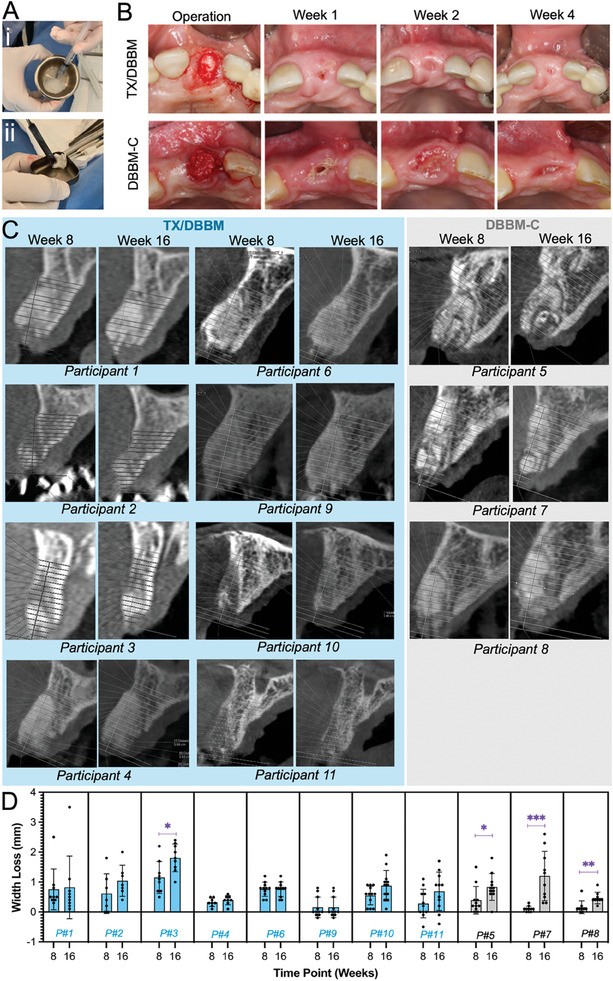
Clinical study of TX/DBBM in ARP post tooth extraction. A) TX/DBBM sample preparation before the operation: i) mixing TX hydrogel with DBBM, and ii) forming a moldable and cohesive putty of TX/DBBM. B) Treatment site at operation (Day 0), and Week 1, 2, and 4 post‐operations of participants with TX/DBBM (TX/ Bio‐Oss®) or DBBM‐C (Bio‐Oss® Collagen) as the Control. Completion of Mucograft ®Seal and tissue epithelization were used to assess the rate and extent of wound closure in participants. C) CT scans of the socket sites treated with TX/DBBM and DBBM‐C at 8 and 16 weeks post‐treatment, the increments (dotted lines) are sliced at 1 mm D) Width loss in the region of interest of the treated sites of all participants treated with TX/DBBM (blue) and DBBM‐C (grey). Data are expressed as mean ± SD. A two‐tailed *t*‐test was performed on means of 8 weeks versus 16 weeks for each patient and the statistical significance was demonstrated as follows: P* < 0.05, *P* < 0.01, P* < 0.001.
